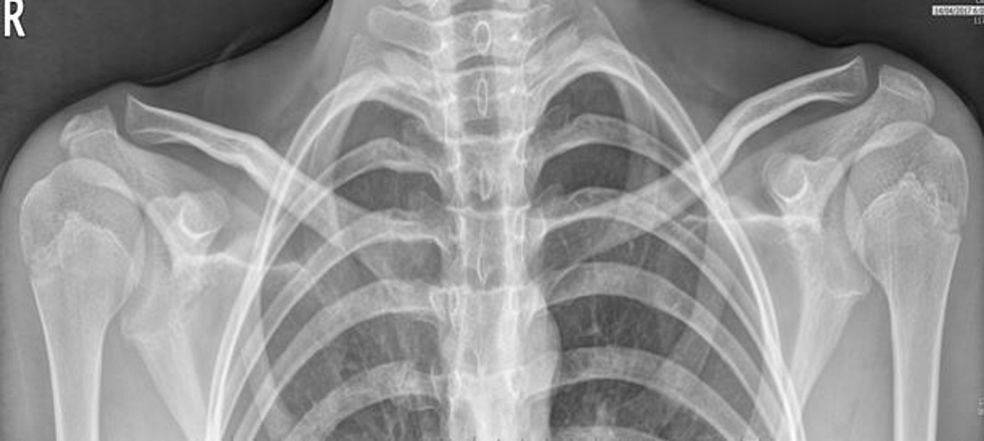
X-ray of the sternoclavicular joints The image displays the normal anatomical appearance of sternoclavicular joints and visualized osseous structures
